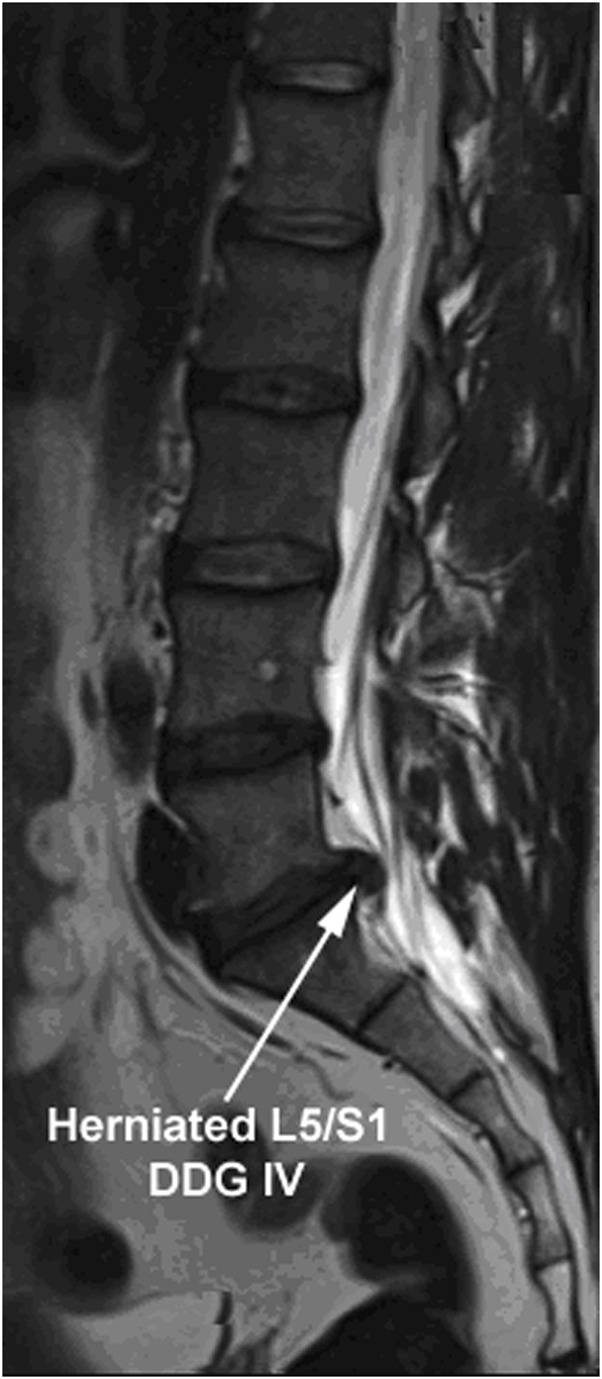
T2-weighted MRI image representing the herniated IVDs of DDG IV. T2-weighted MRI image demonstrating a herniated lumbar IVD (L5/S1) of disc degeneration grade IV (DDG IV) with nerve root compression that remained unresponsive to non-operative treatment for 6 weeks. Sixteen patients harboring a herniated lumbar IVD of DDG IV were involved in this study (age range: 40–69 years, mean age: 55 years). The MRI based interobserver reliability agreement for rating of DDG was ascertained with 100% frequency of agreement (κ = 1.00).
